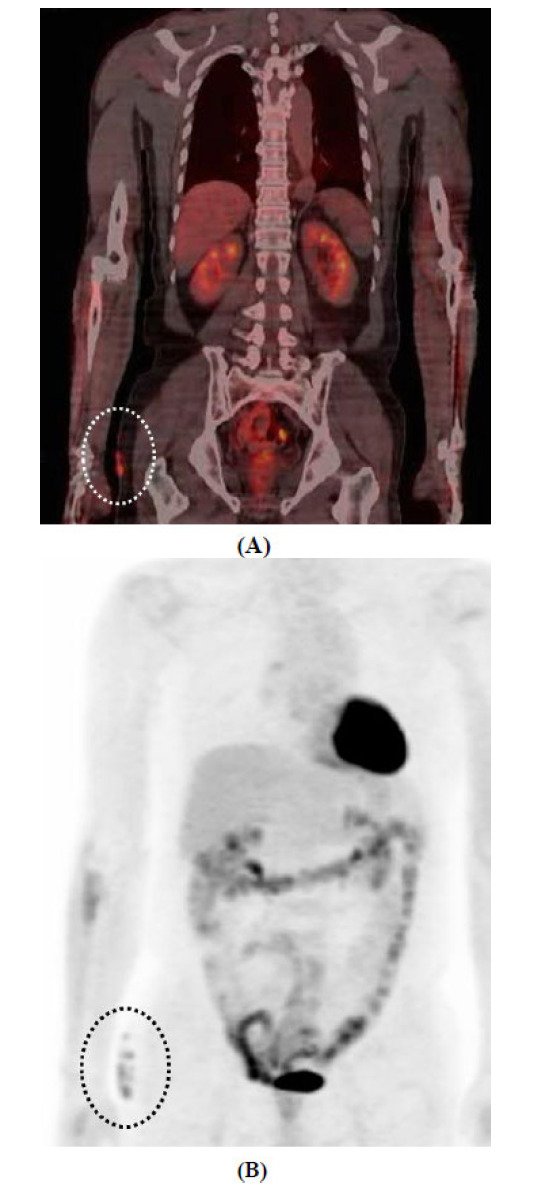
(A) Fused PET/CT image with a white dotted circle highlights the clustered hypermetabolic nodules in the dermal layer of the right pelvis. (B) Maximum intensity projection (MIP) PET/CT image with a black dotted circle demonstrates the corresponding hypermetabolic lesions.
